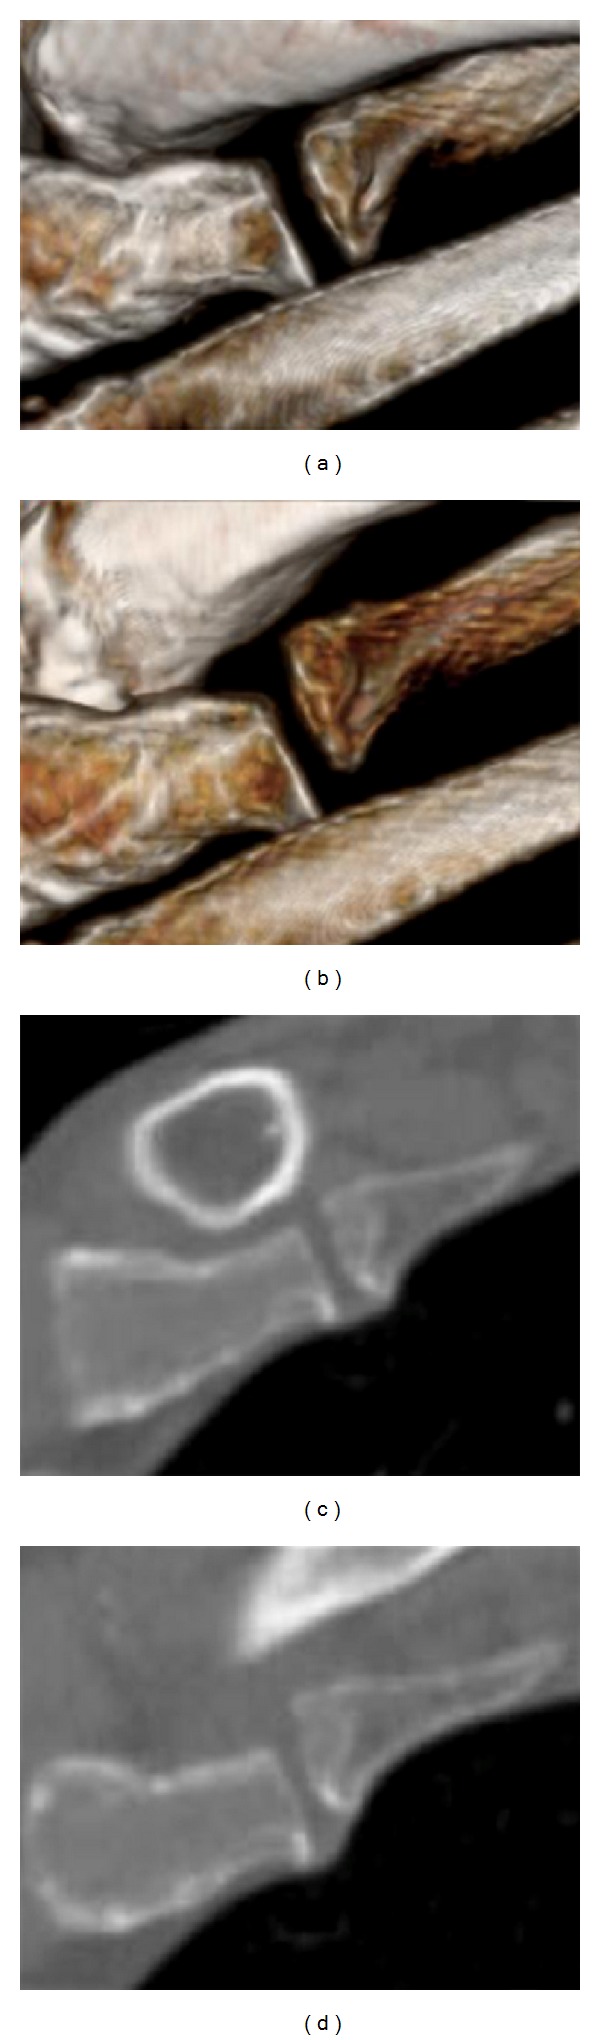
Computed tomography (CT) images of the left first sternocostal synchondrosis. Three-dimensional reconstruction (a, b) and coronal CT images (c, d) showing subluxation of the left first rib in two different positions, with the arm hanging naturally (a, c) and elevated (b, d).
